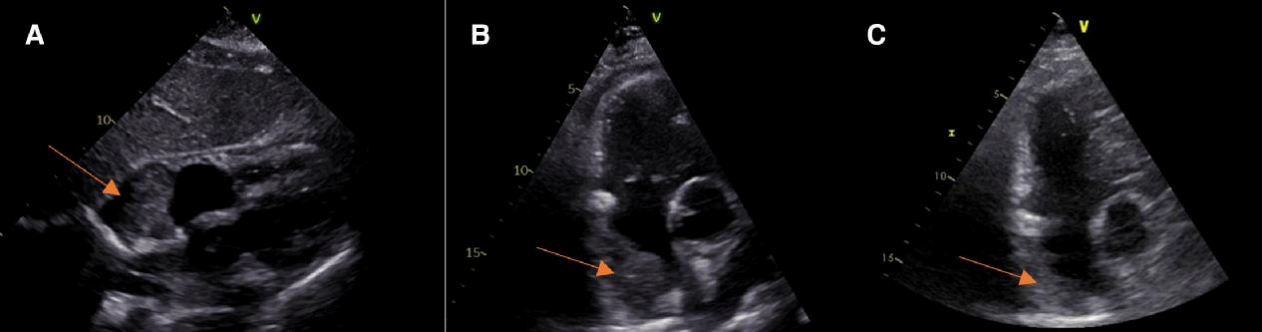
Transthoracic echocardiogram with subcostal plane (*A*) and short axis view (*B*) demonstrating the large right atrial mass (arrows) in the area of the right atrium with inhomogenous tissue contrast and smooth borders. (*C*) Showing the size regression of the mass in follow-up in short axis view.
